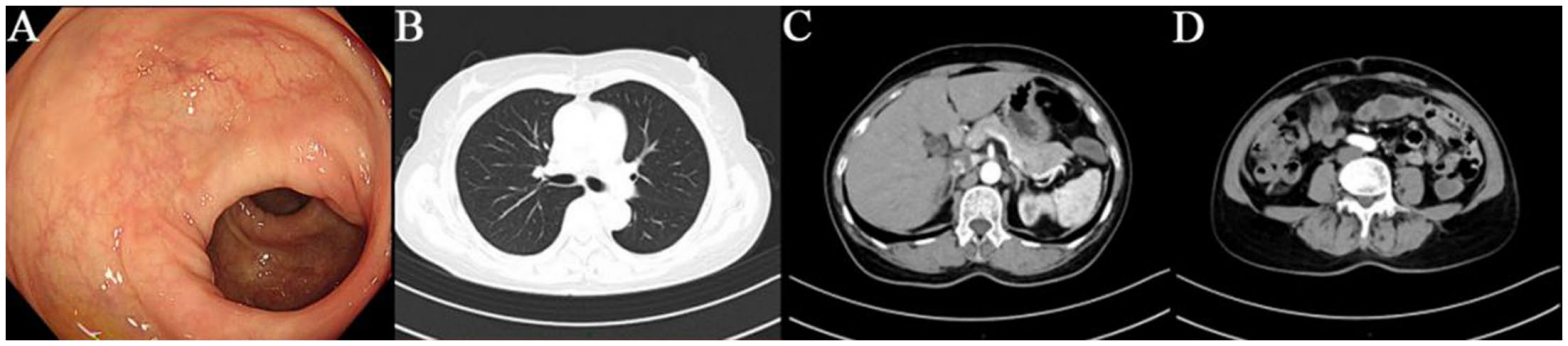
(A) Follow-up colonoscopy showed smooth and unremarkable mucosa. (B–D) Follow-up chest computed tomography (CT) and abdominal CT revealed no signs of recurrence or metastasis.
